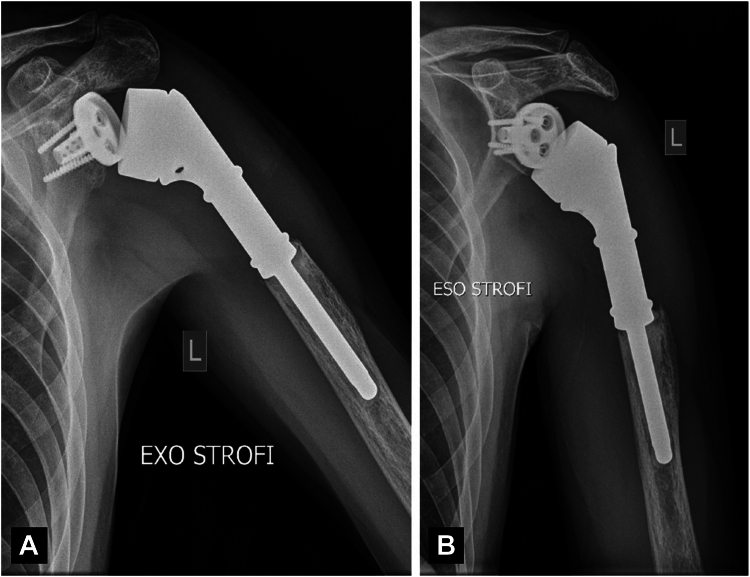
(A, B) Follow-up X-rays at 6 months postoperatively, showing normal alignment of the prosthesis without evidence of loosening
